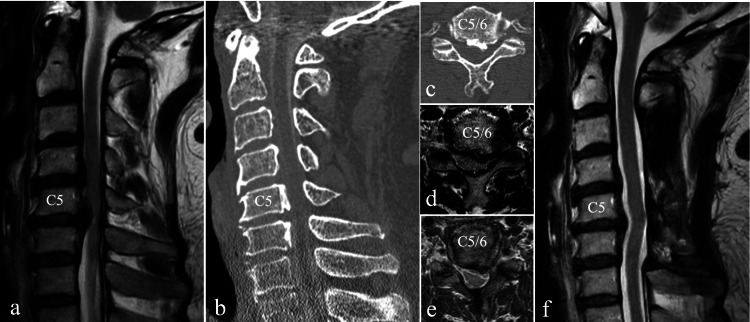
Preoperative MRI (a, d), CT (b, c), and postoperative MRI (e, f) of case 1 a. Preoperative MRI T2-weighted sagittal section showed the spinal canal stenosis from C3 to C6, with severe spinal cord compression at C5/6. b. Preoperative CT myelography sagittal section showed the ossification of the posterior longitudinal ligament at C4 to C6. c. Preoperative CT myelography transverse section of C5/6 showed compression of the left C6 nerve root due to the ossification of the posterior longitudinal ligament. d. Preoperative MRI T2-weighted transverse section of C5/6 showed compression of the left C6 nerve root and spinal canal stenosis. e. Postoperative MRI T2-weighted transverse image showed that the left C6 root and spinal canal were decompressed by laminectomy and foraminotomy. f. Postoperative MRI T2-weighted sagittal section showed that the spinal cord was decompressed.
